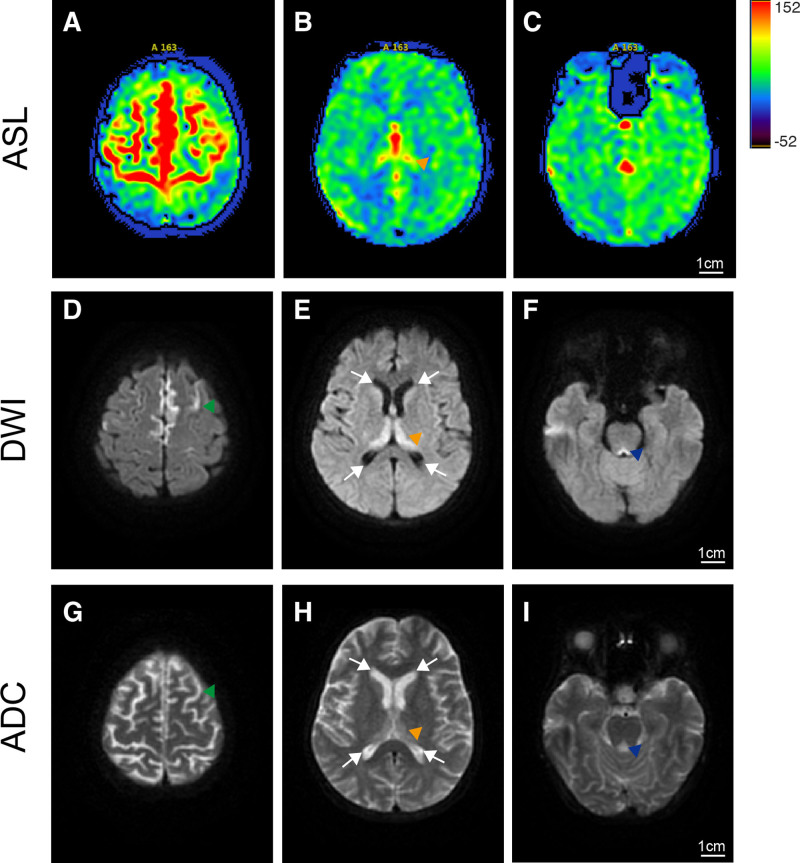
(A to I) MRI images acquired on the 10th day post admission. (A) Arterial spin labeling (ASL) revealed cerebral blood flow (CBF) increased in large areas of the bilateral frontal and parietal lobes beside the midline. Concurrently, peripheral cortical perfusion was insufficient. (B and C) ASL showed high CBF in the internal bilateral thalamus (yellow triangle) and the periaqueductal area (blue triangle) at the posterior margin of the brainstem. (D, E, and F) DWI demonstrated high signal intensity in surrounding areas of the midline of the bilateral frontal and parietal lobes (green triangle), the internal bilateral thalamus, bilateral caudate nucleus head (white arrows), and the periaqueductal area at the posterior margin of the brainstem. (G, H, and I) did not show clearly changes in the same regions. Scale bar = 1 cm. DWI = diffusion-weighted imaging, MRI = magnetic resonance imaging.
